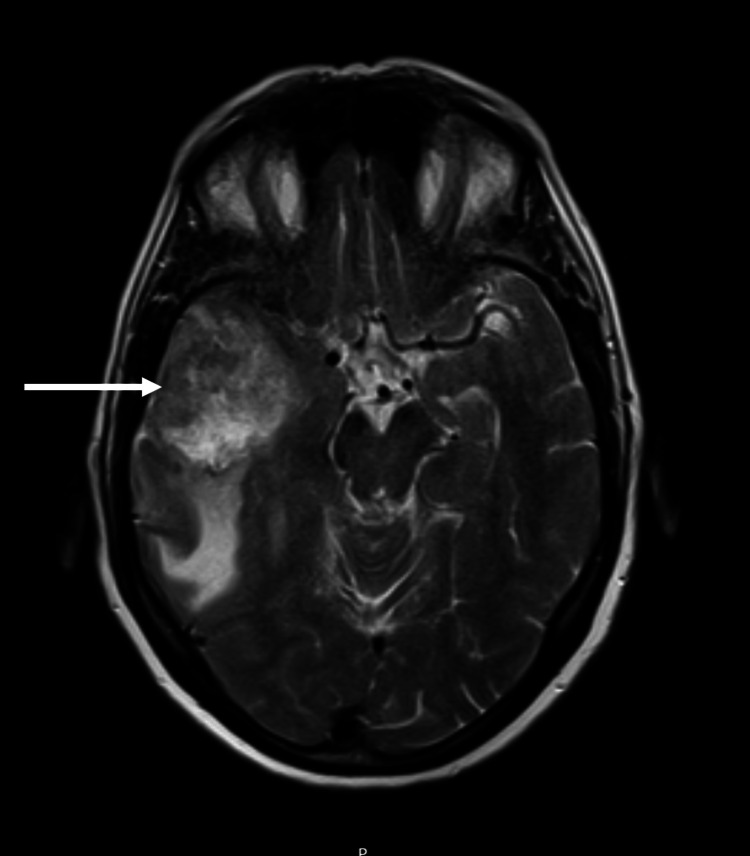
MRI showing a right frontal temporal lesion measuring 43 × 38 × 43.5 mm.
